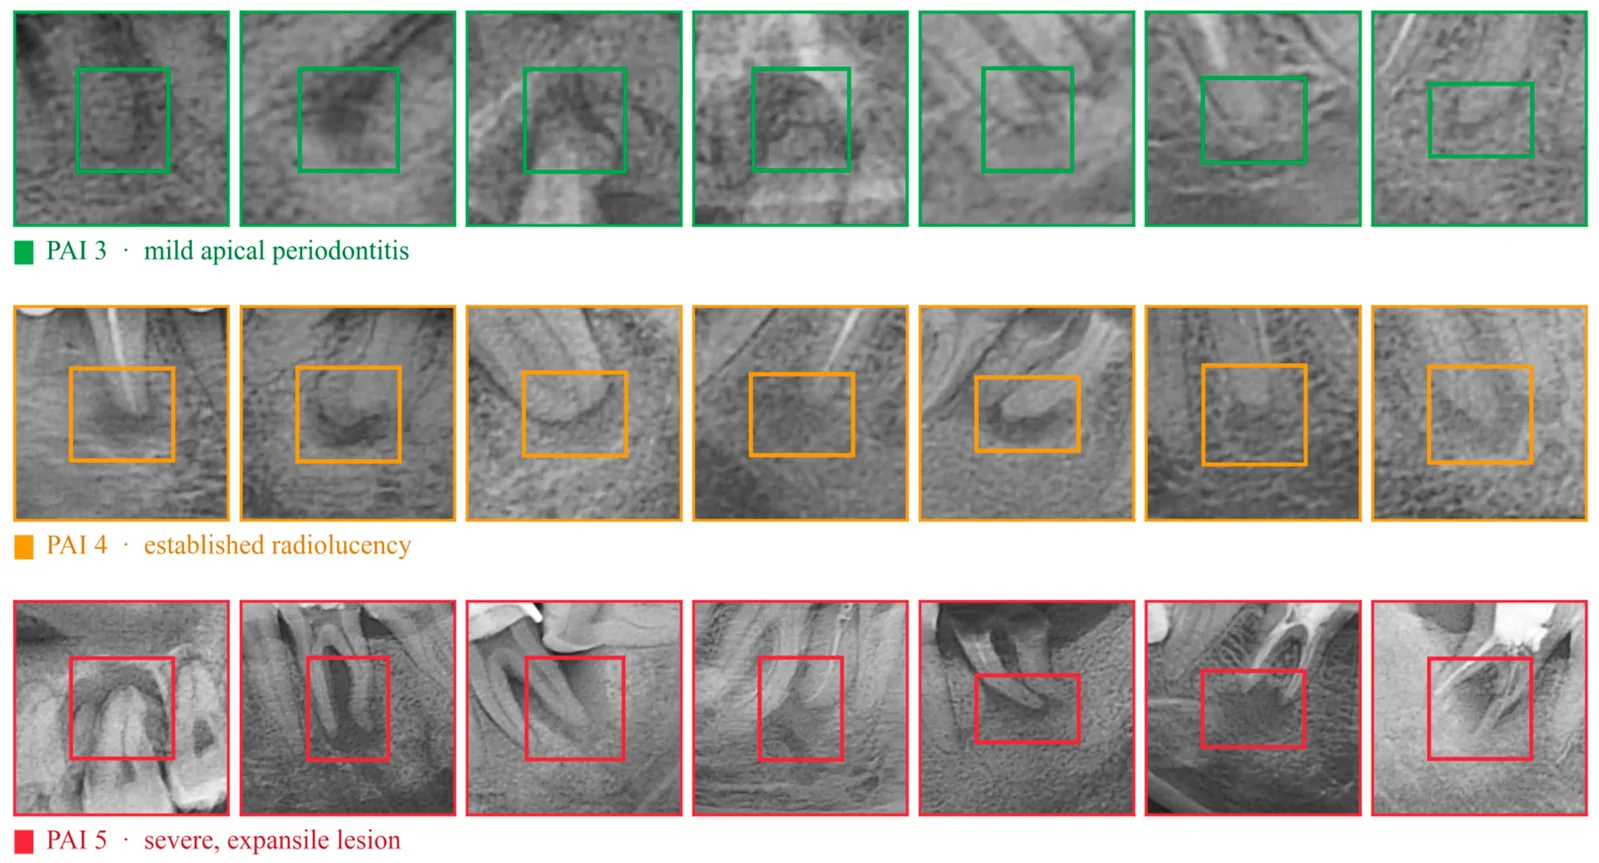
Representative periapical lesions for each grade of the PAI. Each row collects seven examples of a single grade, cropped from the native-resolution radiograph as a square centred on the annotated bounding box whose side is 2.1 times the larger side of that box, so that the surrounding anatomy is visible, and then scaled to a common panel size, with the box drawn in the colour of its grade. A typical lesion box has a median side of 60 pixels with an interquartile range of 50 to 77, so a typical crop covers about 126 pixels of the original radiograph and the panels are not all at the same magnification.
